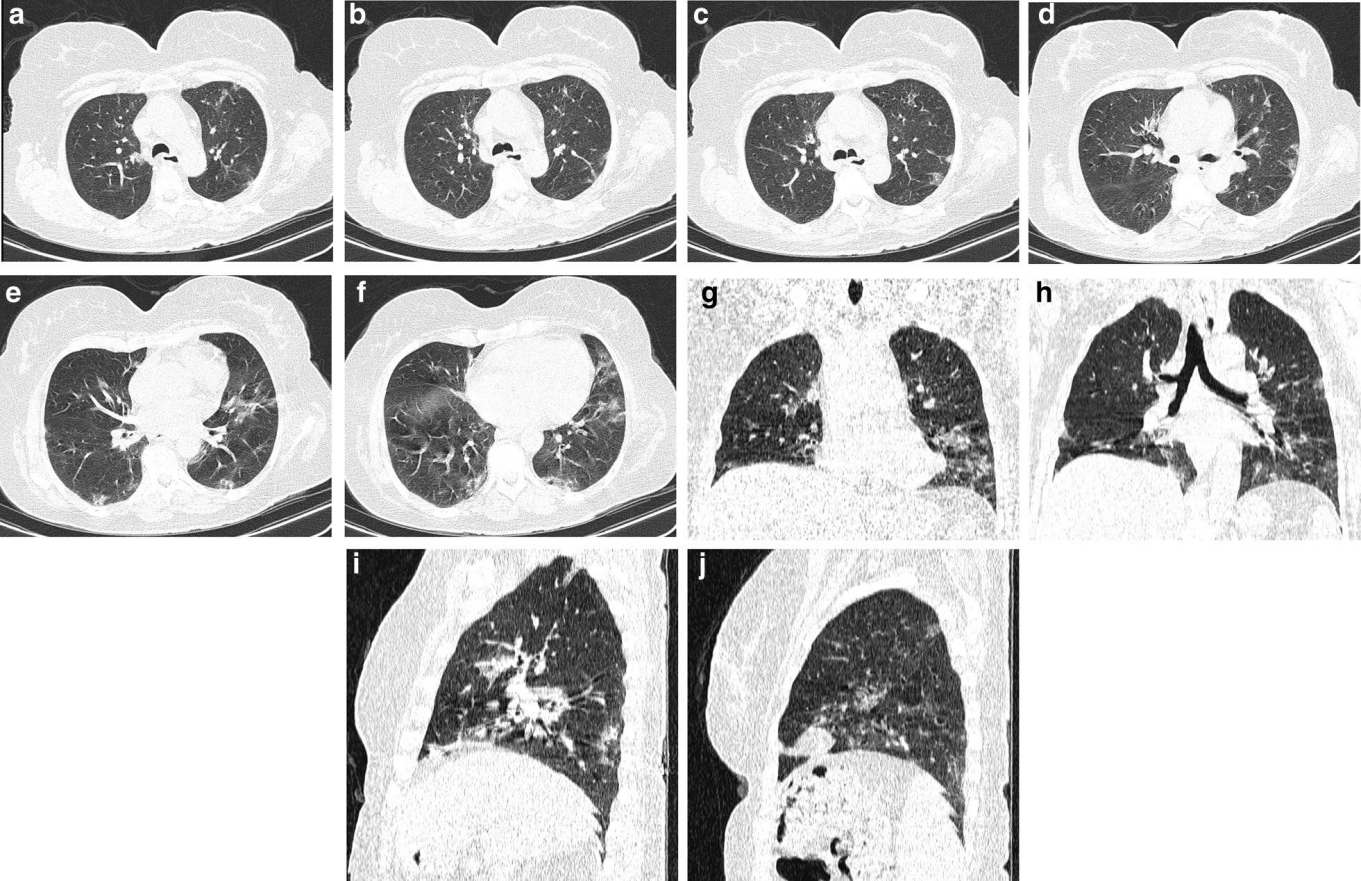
A 62-year-old, non-diabetic lady without any identifiable risk factor showed some lung involvement in her first-day in-hospital CT (A–J) CTSS1 = 8/24, CTSS2 = 14/48, CTSS3 = 6/20, CTSS4 = 12/25, CTSS5 = 10/20, CTSS6 = 17/40, CTSS7 = 14/30. After admission, she stayed in the ward for 6 days (moderate disease on triage), and then, disease aggravation led to admission in ICU (severe disease on prognostication); she was not intubated and was discharged after staying 7 days in ICU
